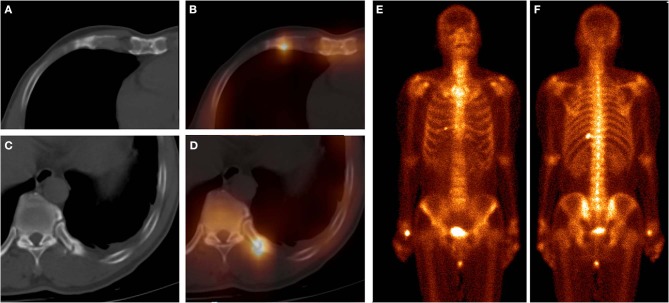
These images were obtained from a 61-year-old man with atraumatic CCF of SG, who underwent chemotherapy for left lung squamous cell carcinoma 5 months ago. ^99m^Tc-MDP SPECT/CT axial images (A, CT; B, fusion; C, CT; D, fusion) and WBS image (E, anterior; F, posterior) demonstrated high-uptake tracer activity in the calcification of the right fifth costal cartilage, with local deformation. Moreover, another focus of intense tracer was noted in the left 11th posterior rib, with fracture line and callus. These indicated that this patient was at a high risk of fracture.
